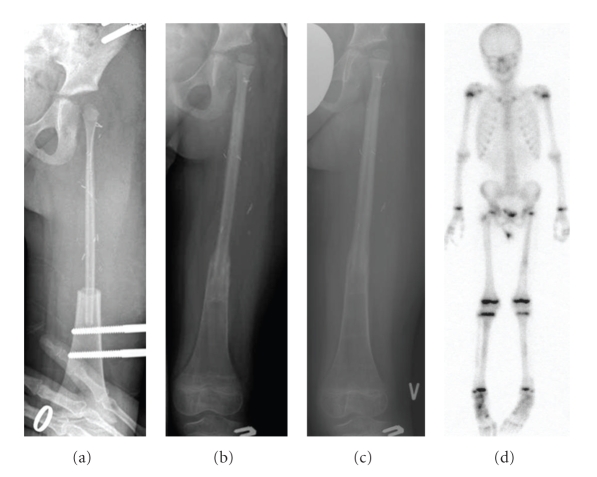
Resection of the proximal part of the femur because of Ewing's sarcoma and reconstruction with a vascularized fibula graft including the proximal epiphysis (patient 2). X-rays taken 2 weeks postoperatively (a), with follow-up after 7 months when weight-bearing was started (b), after 12 months when full weight-bearing had been allowed for 3 months (c), and finally a bone scan performed 12 months postoperatively (d).
